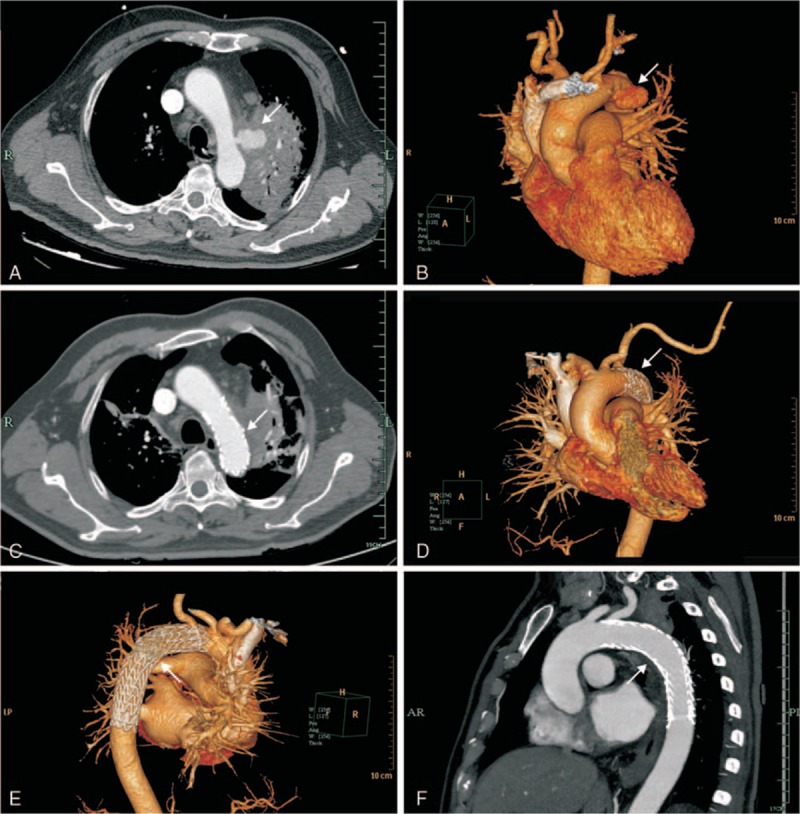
Preoperative CTA: thoracic CT scan showing an irregular wall at the level of the aortic arch, which is an aortic arch pseudoaneurysm (A, B). Follow-up CTA: CTA on the 5th postoperative day showed the shape and the location of the stent-graft were satisfactory, and no evidence of endoleak, filling, or enlargement of the pseudoaneurysm (C–F). CT = computed tomography, CTA = computed tomography angiography.
